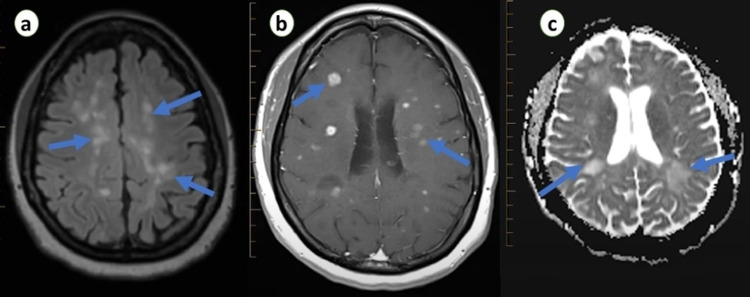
Transverse section of brain MRI showing white matter (a), juxtacortical (b), and paraventricular (c), deep lesions in various stages of enhancement in both hemispheres.
